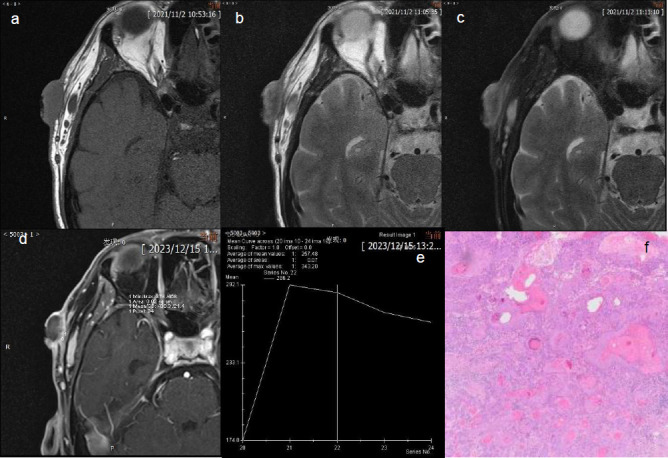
MRI of SCC in right temporal area. The tumor appears isointense on T1WI (a), asymmetric hyperintensity on T2WI (b) and asymmetric hyperintensity on FST2WI (c). The dynamic enhancement imaging showed ring enhancenment pattern with ulcer (d). The dynamic enhancement curve showed an outflow pattern (e). The pathology confirmed SCC (10×) (f).
